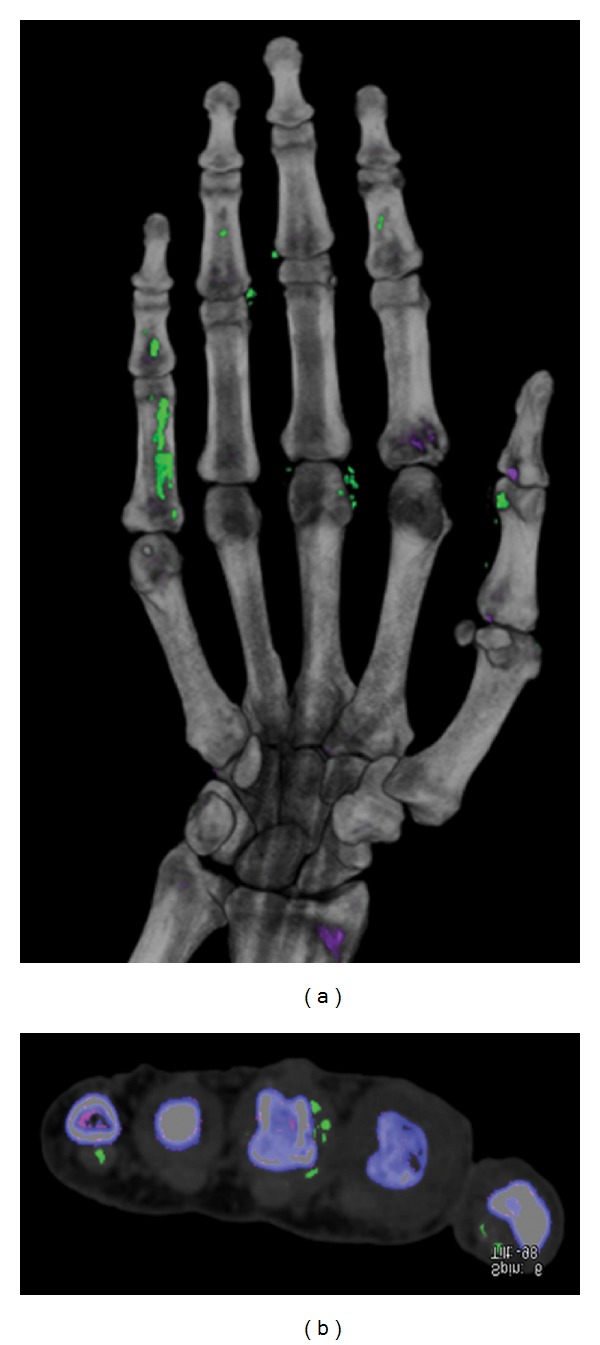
Dual energy computed tomography (DECT) images (a, b) of a hand showing tendinous and periarticular MSU deposition (color coded—green). (Courtesy Dr. K. Glazebrook, Mayo clinic, Rochester, MN, USA).
